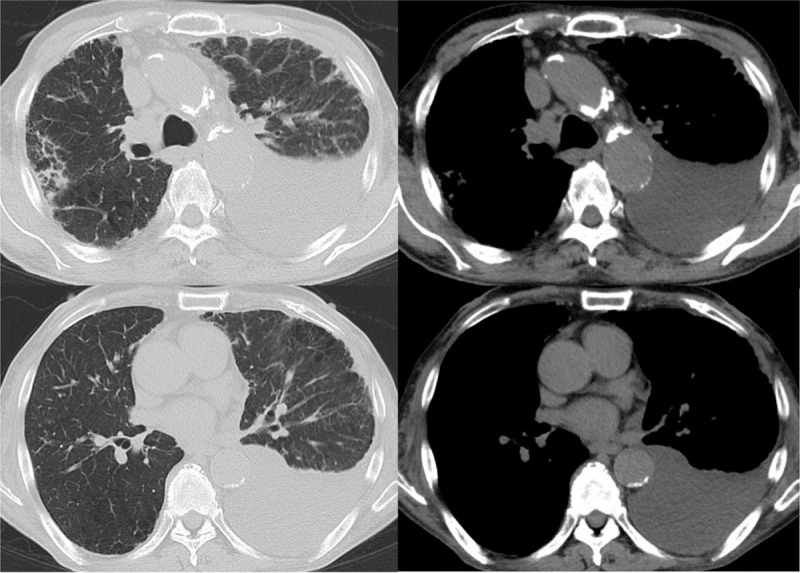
A chest high-resolution computed tomography scan. Chest CT showed a moderate amount of left pleural effusion, peripheral consolidation with interlobular septal thickening in mainly the bilateral upper lobe, emphysema, and swelling of the mediastinal lymph nodes.
